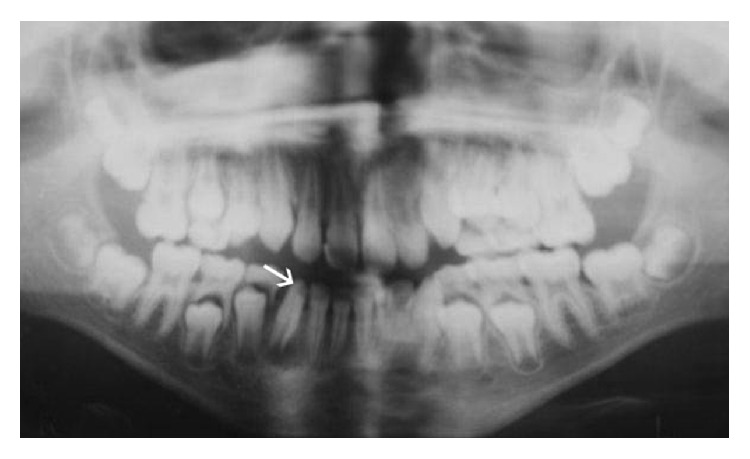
Orthopantomogram taken at initial presentation showing periapical changes in the LR3.
